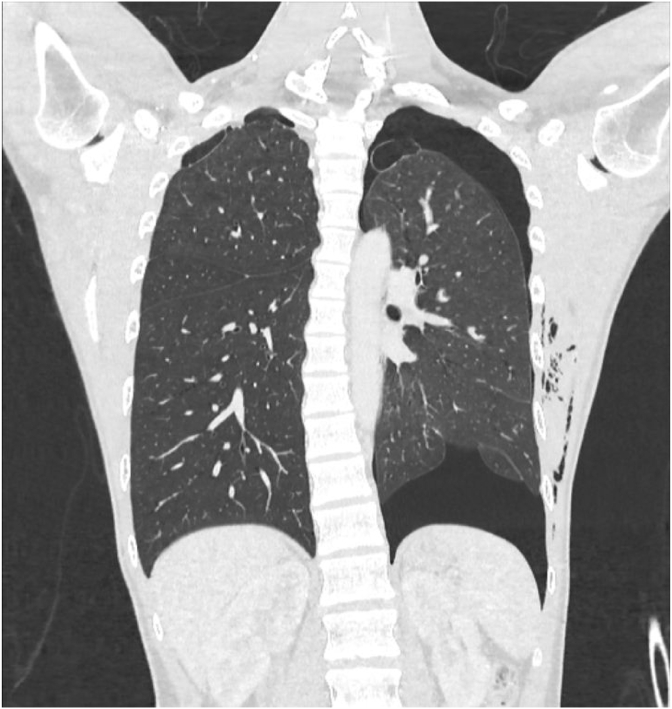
CT Thorax coronal view displaying left pneumothorax with subpleural bullae over apical region of bilateral upper lobes.
